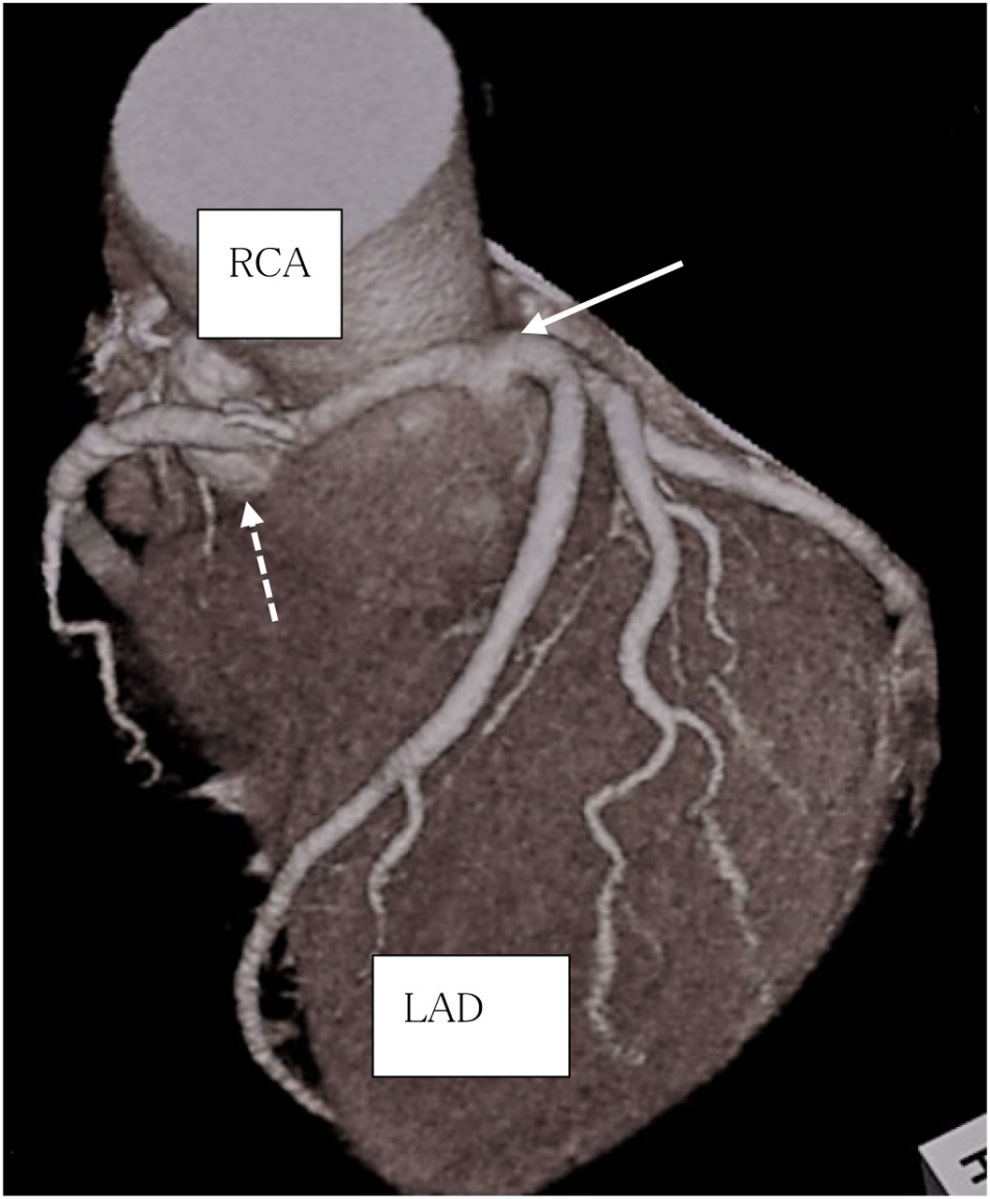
Preoperative CT. (1) right coronary artery originates from the left coronary artery(←). (2) abscess cavity is in contact with the RCA (#1). LAD, left anterior descending arteryl; RCA, right coronary artery.
